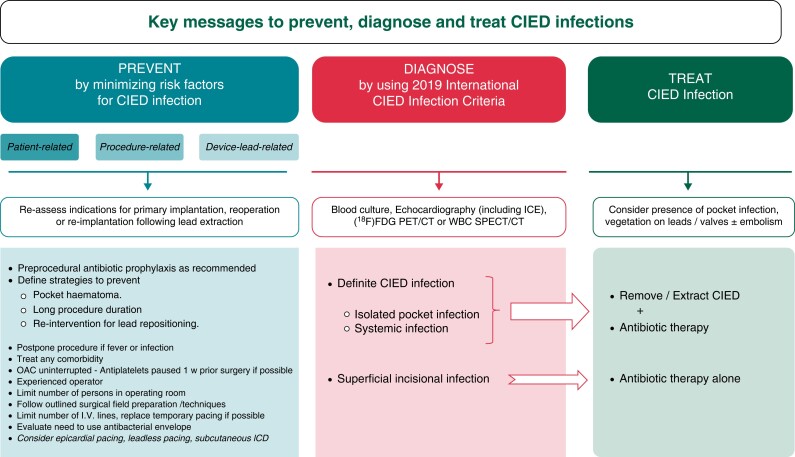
Summary of key messages for prevention, diagnosis, and management of CIED infections. From ref.^71^ CIED, cardiac implantable electronic device; [18F] FDG PET/CT, fluorodeoxyglucose positron emission tomography–computed tomography; ICD, implantable cardiac defibrillator; ICE, intracardiac echocardiography; OAC, oral anticoagulation; w, week; WBC SPECT/CT, white blood cell single-photon emission computed tomography–computed tomography.
